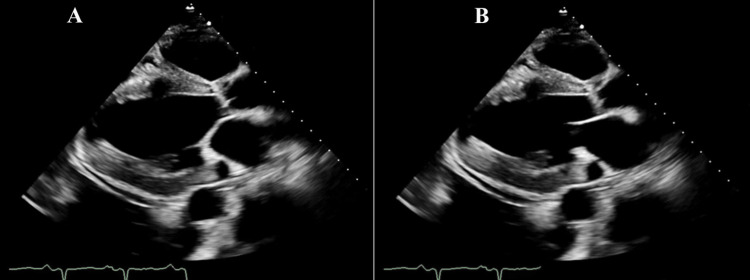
On transthoracic echocardiogram, parasternal long-axis views of the heart in end-systole (A) and end-diastole (B) show dilation and hypertrophy of the left ventricle as well as left atrial dilation. There is a small difference between the diameter of the left ventricle at the end of systole (A) and the end of diastole (B) indicating global left ventricular hypokinesia.
